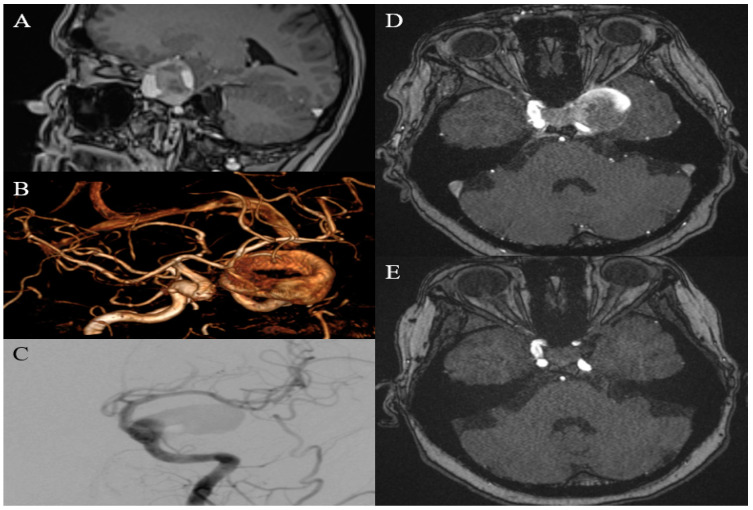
(A) MRI revealed a giant, partially thrombosed aneurysm within the cavernous segment of the left ICA with circular flow and “apple core” shaped thrombus in the aneurysmal sac; (B) MRI–TOF-3D reconstruction shows donut shape of the aneurysm; (C) DSA following the flow-diverter implantation, AP projection; (D) MRI before endovascular intervention; (E) Follow-up MRI after 12 months.
